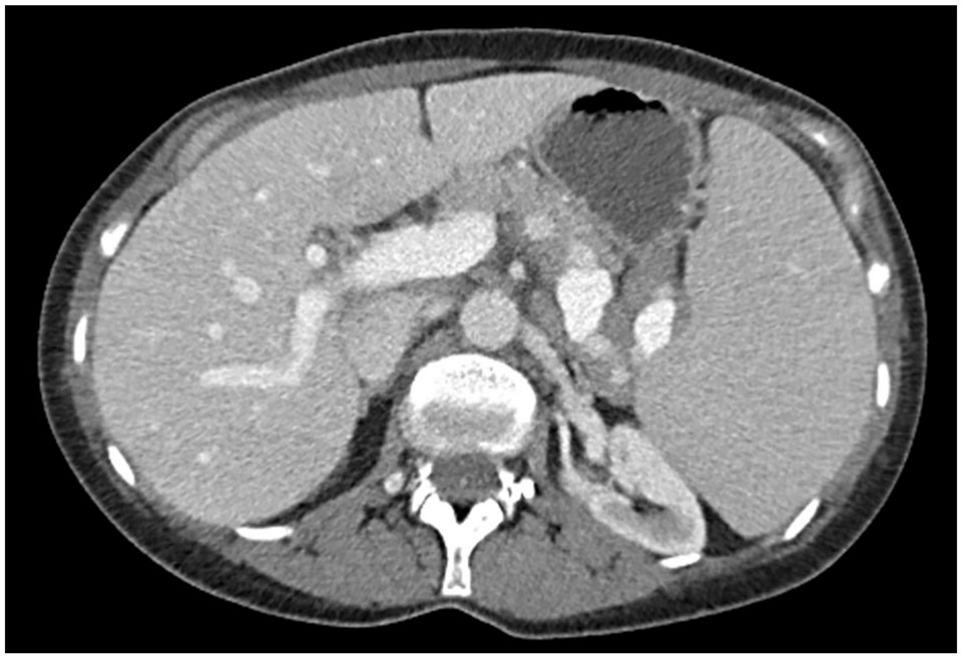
The patient’s abdominal CT scan demonstrates massive splenomegaly with adjacent abdominal lymphadenopathy.
